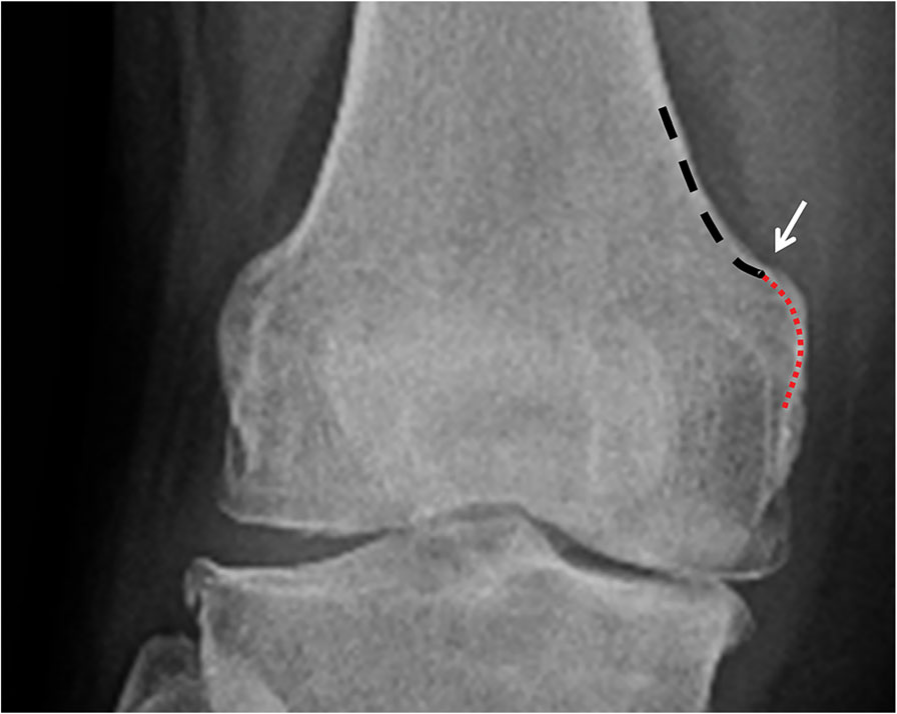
Radiograph showing the inflection point (indicated by the arrow) at the shaft-condylar junction of the distal femur. This point is formed by the juncture of a proximal concave curve (depicted by the coarse arc) and a distal convex curve (depicted by the fine arc)
